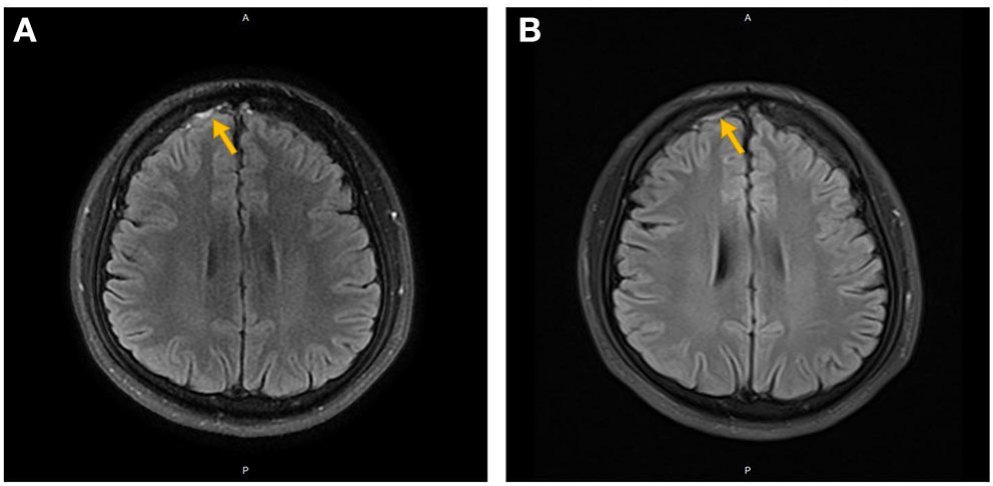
Non-enhanced (A) and contrast-enhanced (B) MRI of the brain in Case 4. The orange arrow indicates subarachnoid hemorrhage in the right frontal lobe.
